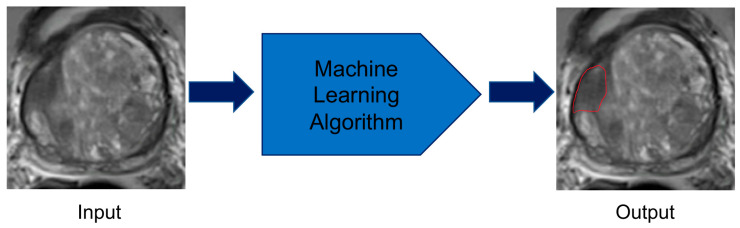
Prostate lesion segmentation using machine learning techniques. The computer takes multiparametric magnetic resonance imaging images of the prostate as inputs and applies the developed machine learning algorithm to correctly identify the borders of the lesion.
